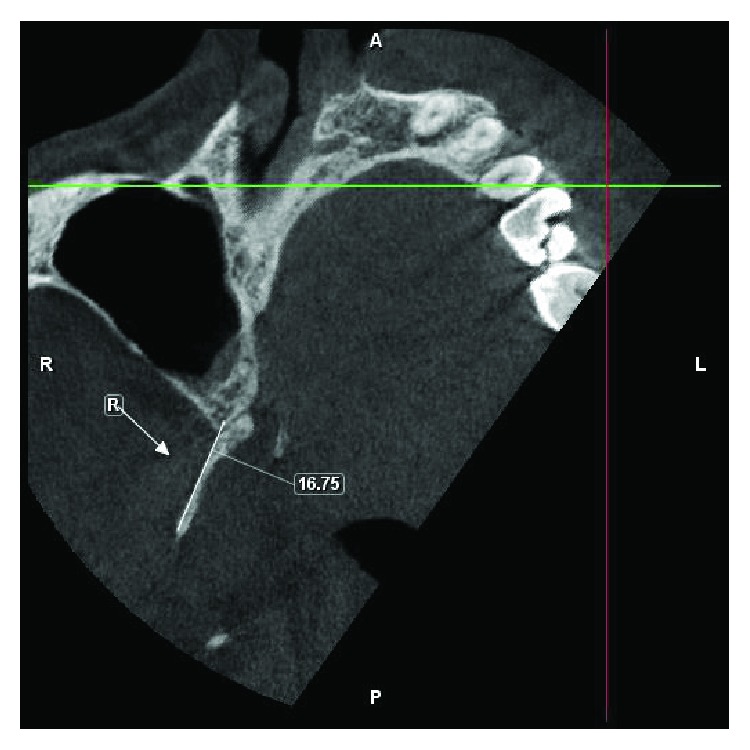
Cone beam computed tomography axial view showing an elongated pterygoid hamulus.
